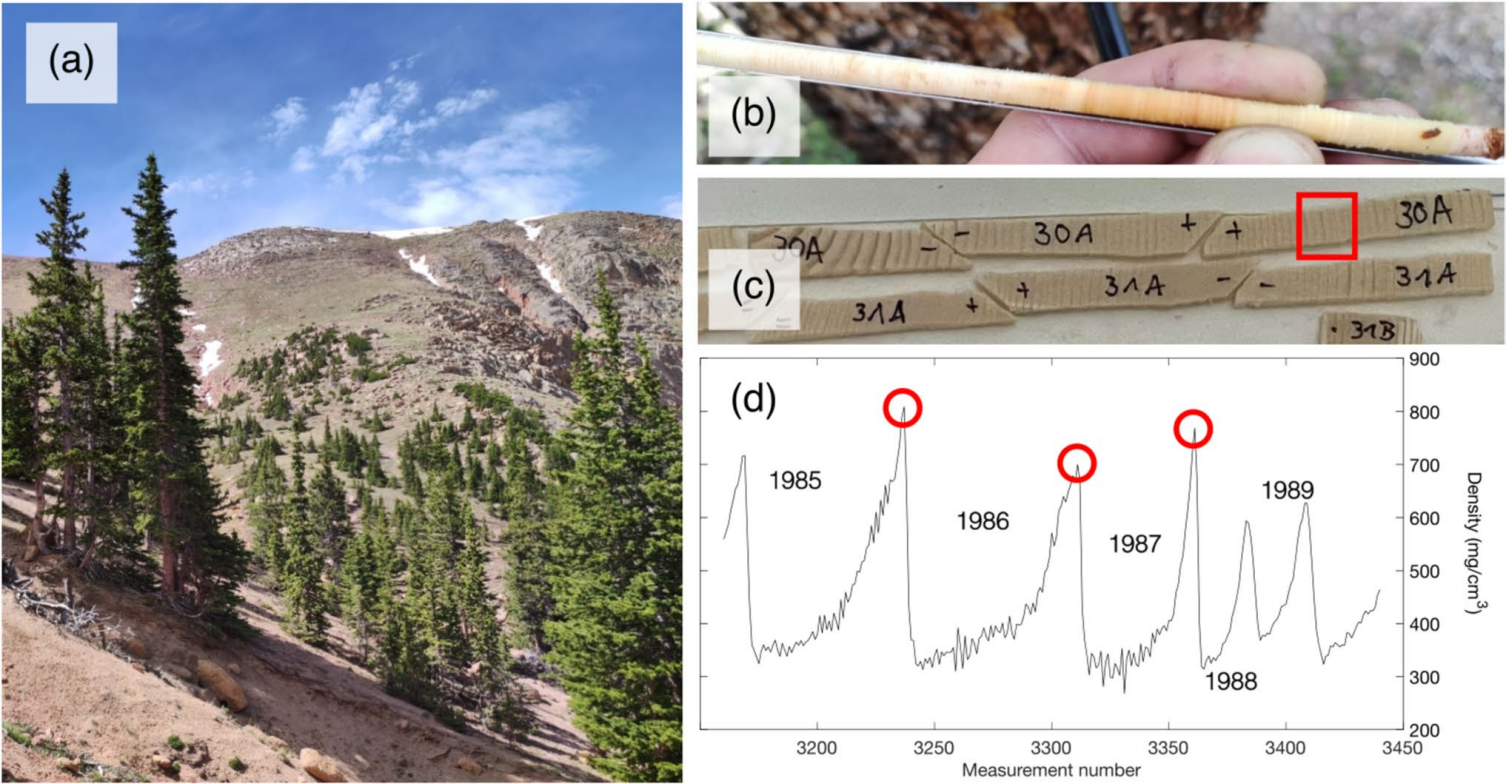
a Photograph of *Picea engelmannii* trees at the sampling site in Pike National Forest, Colorado. b Increment core sample from PNF. c Samples of wood laths prepared for X-ray densitometry. Red square indicates years 1985–1989. d Density profiles from five individual growth rings from sample PNF30A. Red circles indicate the MXD value for 1985, 1986, and 1987
